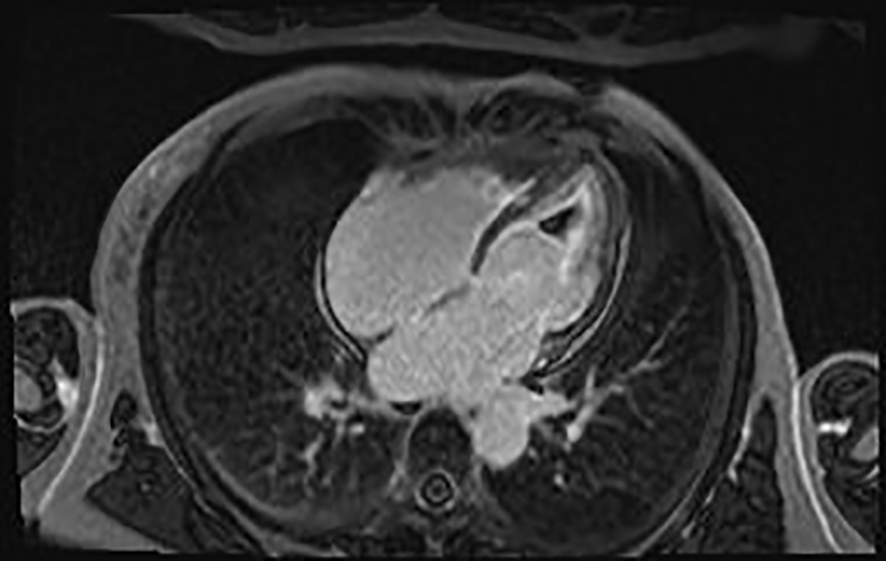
Cardiac MRI with contrast showing diffuse mid to apical left ventricular subendocardial abnormal late gadolinium enhancement.
